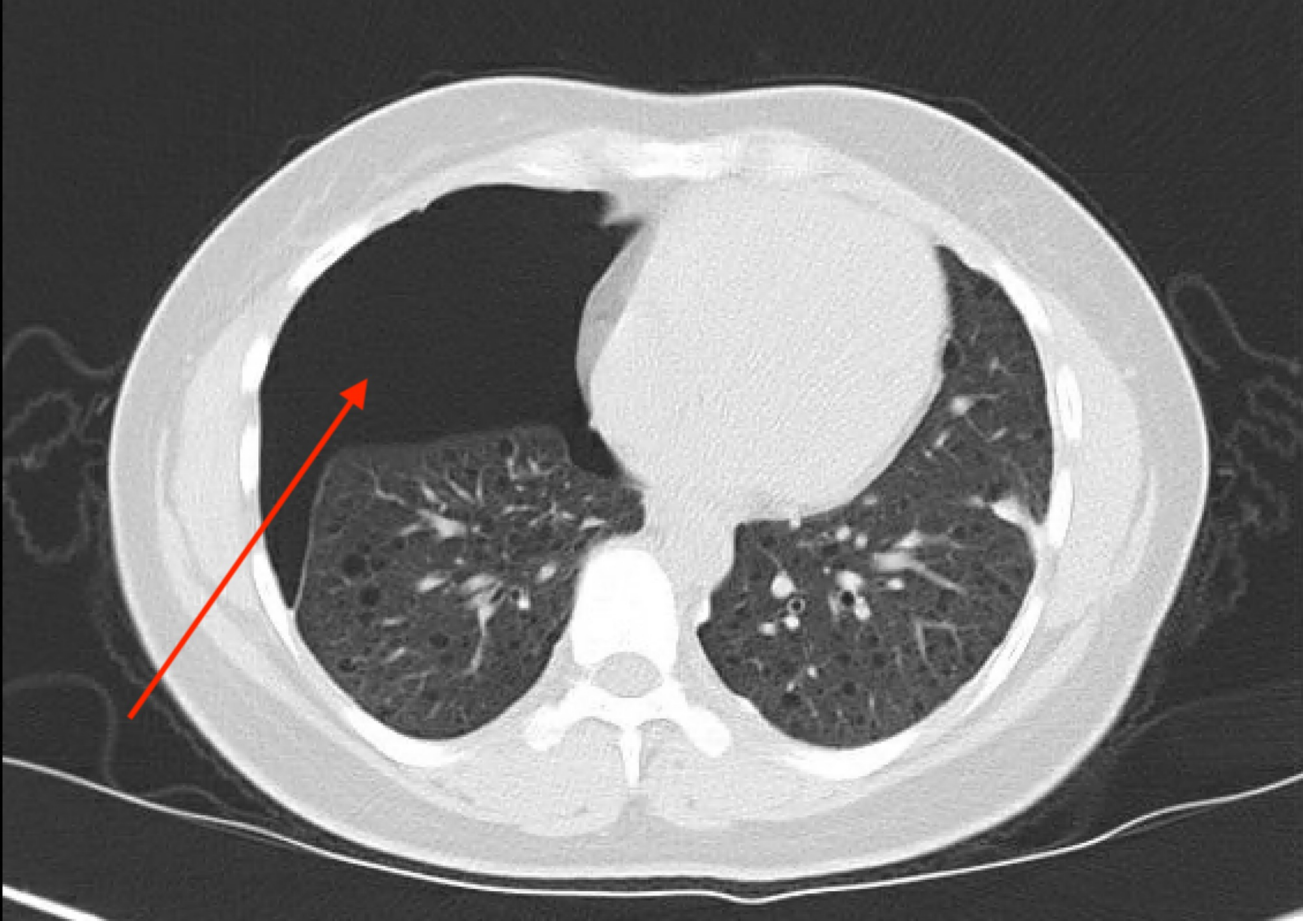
CT of the chest performed on initial visit showing a moderate-sized right anterior pneumothorax (red arrow).
